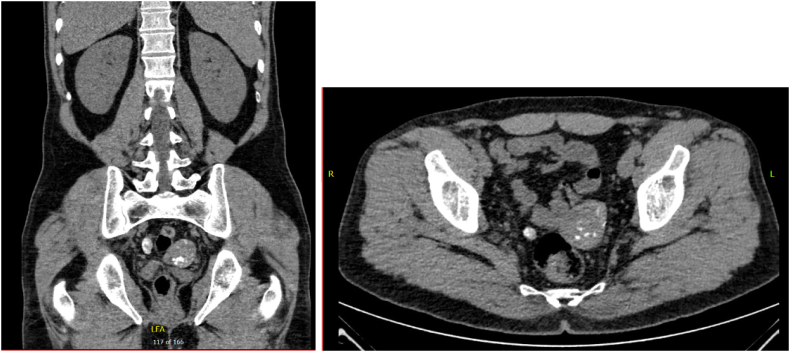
CT showing the 4.9 × 4 cm left pararectal cyst and the 1.5 × 1 cm right pararectal cyst.
